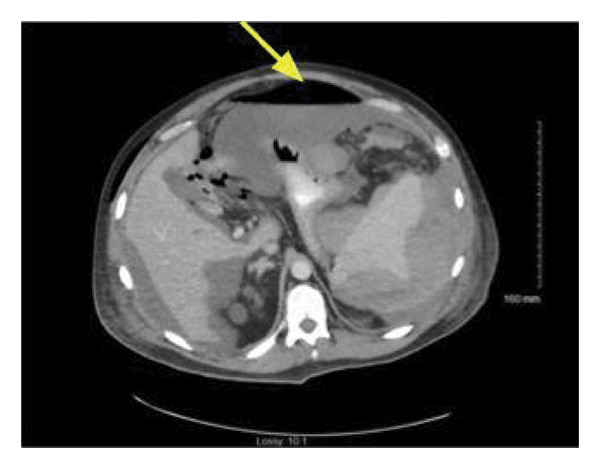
CT abdomen and pelvis with IV contrast shows a moderate volume of intraperitoneal free air mixed with ascites fluid, particularly in the right upper abdomen anteriorly.
